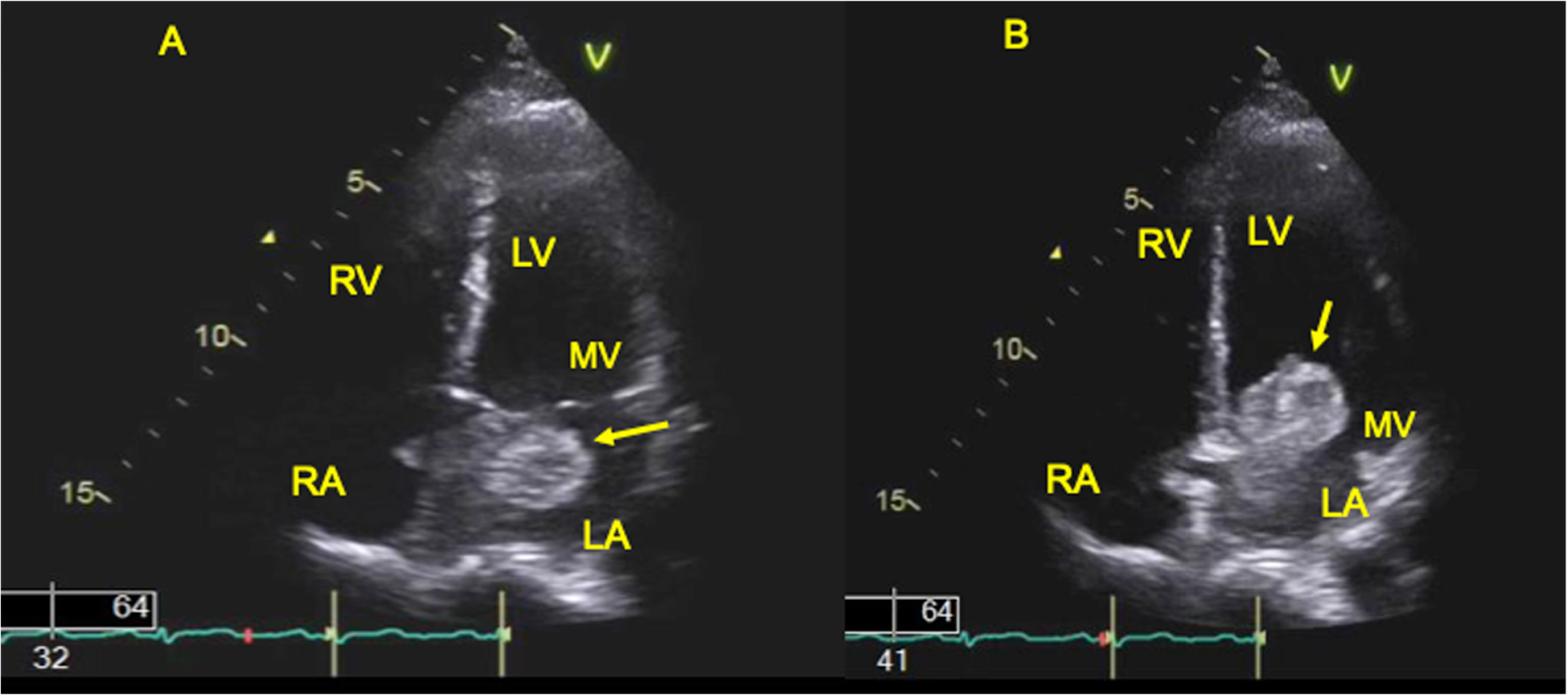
a: Transthoracic echocardiogram (TTE) apical view shows a large left atrial mass at the level of the mitral valve during systole (arrow). Abbreviations: LA-left atrium, LV-left ventricle, RA-right atrium, RV-right ventricle, MV-mitral valve. b: Transthoracic echocardiogram (TTE) apical view, showing the mass at the mitral valve prolapsing into the left ventricle during the diastolic cardiac cycle (arrow). Abbreviations: LA-left atrium, LV-left ventricle, RA-right atrium, RV-right ventricle, MV-mitral valve
